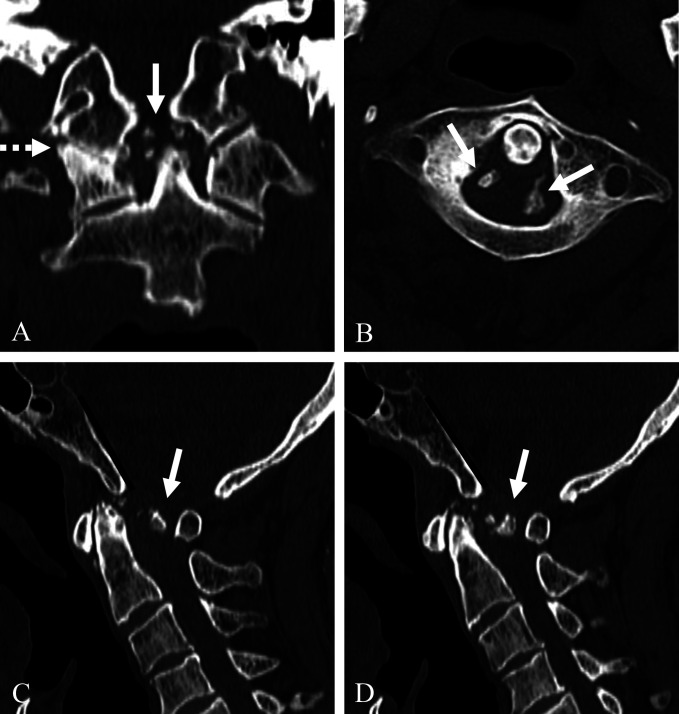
Coronal reconstructed CT (A) scan showing severe osteoarthritic changes (dotted white arrow) and posttraumatic osteophytes (white arrow) of the C0-1 joint. Axial CT (B) scan showing posttraumatic osteophytes of the C1-C2 joint (white arrows). Sagittal reconstructed CT (C and D) scans demonstrating the Wackenheim line (dark lines) behind the dens and posttraumatic osteophytes of the C0-C1-C2 joints (white arrows).
